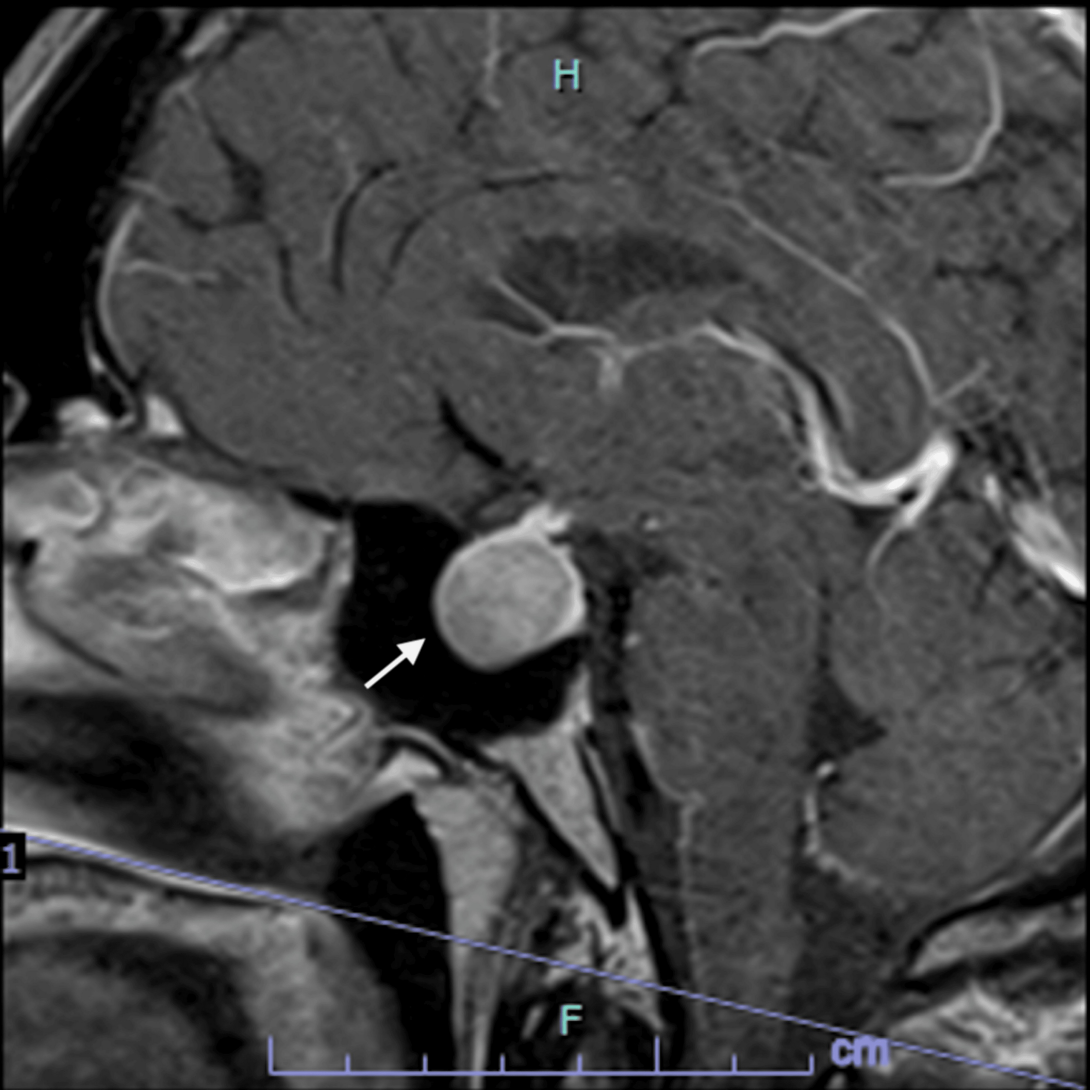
MRI Sella turcica. MRI of the Sella turcica (Coronal T1-weighted image after gadolinium ‍‌‍‍‌‍‌‍‍‌contrast‌‍‍‌‍‌‍‍‌) showing a 17×23×17 mm pituitary macroadenoma extending superiorly to compress the optic chiasm (arrow).
